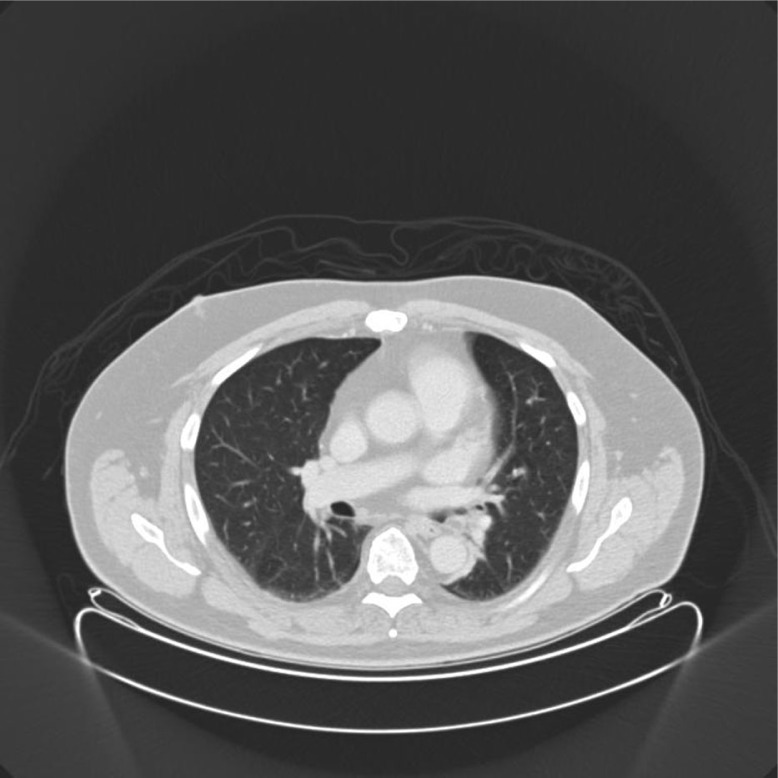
Chest CT before treatment, showing a lesion inside the left bronchial system.
